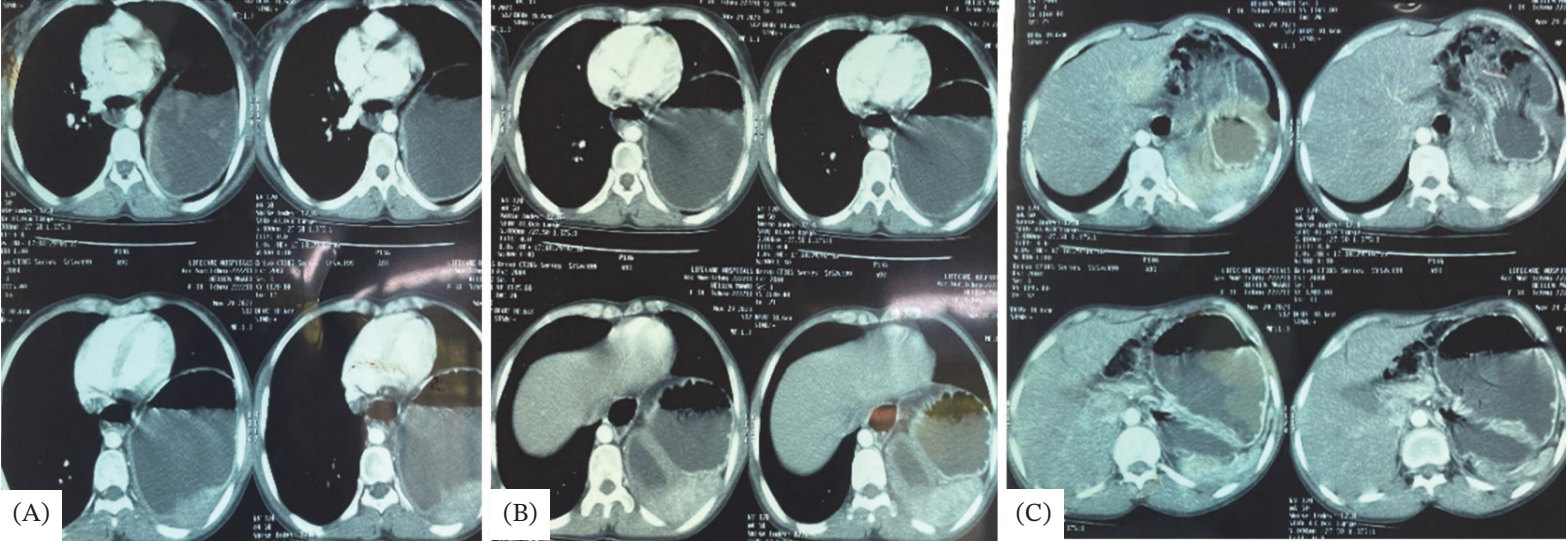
(A) Axial CT scan showing the stomach in the left chest cavity. (B) Shows diaphragmatic constriction. (C) Shows the distal body of the stomach and the antrum.
